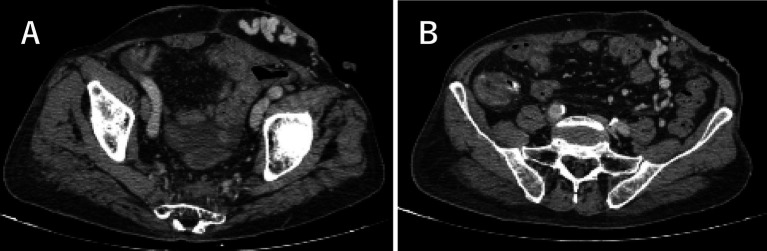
The transverse sections of whole abdominal enhanced CT images. (A) Dilated and tortuous vein shadows visible around the stoma (blue arrow). (B) The main trunk of the varicose vein around the stoma was located in the left branch of the inferior mesenteric vein (red arrow).
